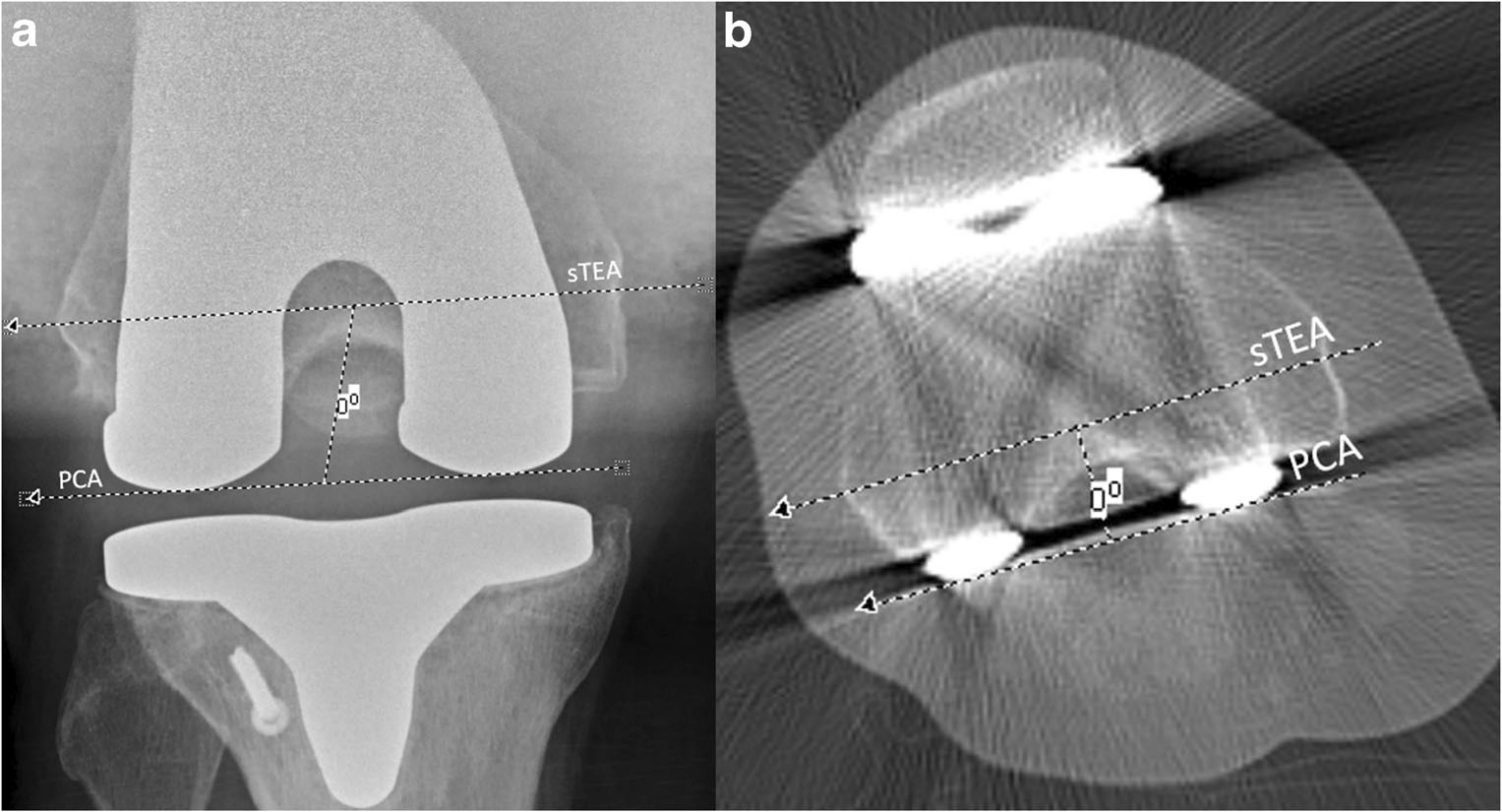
Surgical posterior condylar angle formed by the surgical transepicondylar axis (sTEA) and the posterior condylar axis (PCA) on Kanekasu view radiograph (a) and 2D axial computerised tomography (b) of a 64-year-old man after total knee arthroplasty
